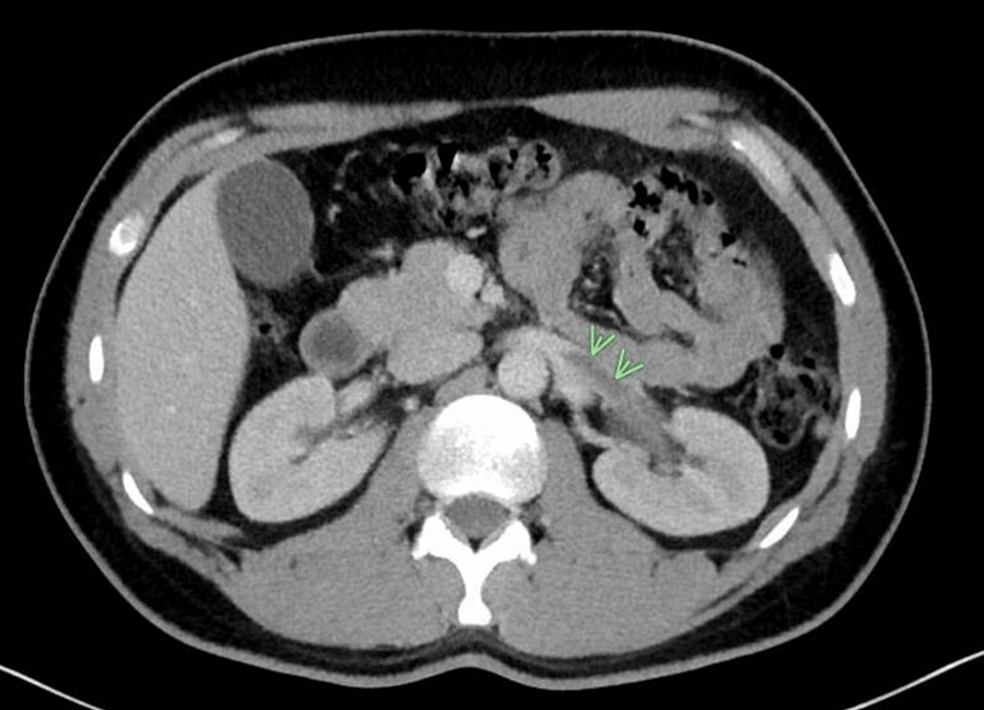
Computed tomography (CT) of abdomen and pelvis with contrast showing thrombosis of the left renal vein (green arrows)
